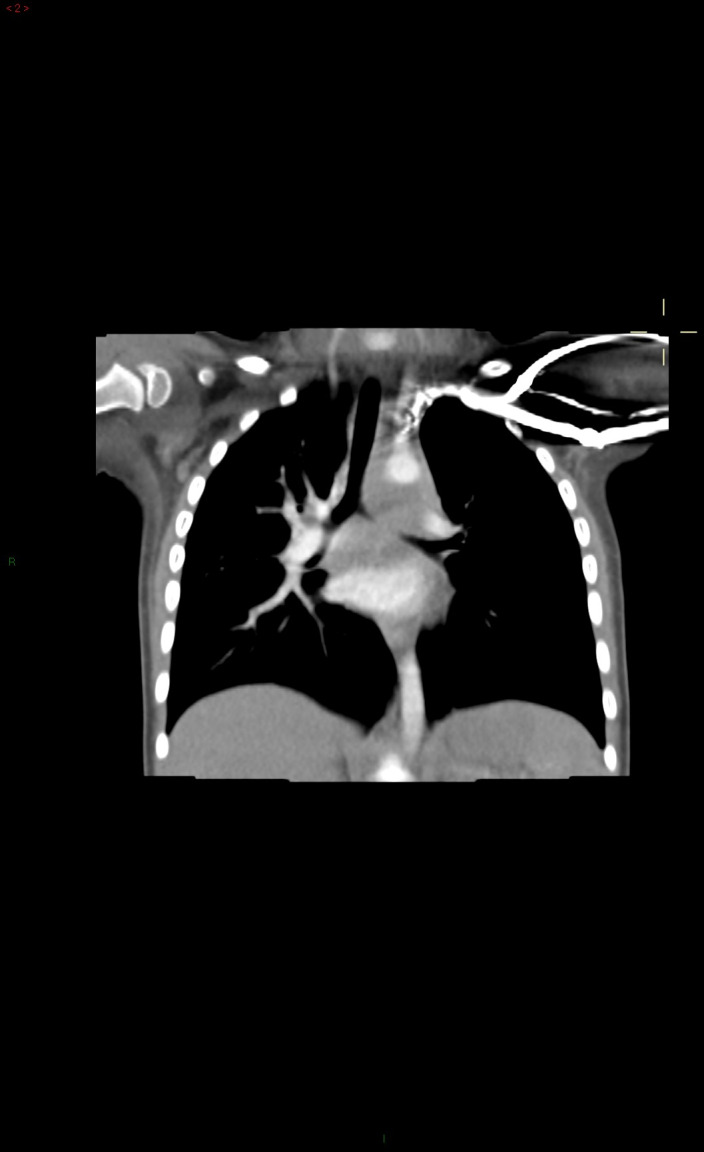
HR‐CT with contrast reveals a large hypodense lesion (34 × 15 × 46 mm) with distinct and smooth margins and fluid density in the posterior mediastinum. This cystic lesion induces the left lung hyperinflation and hypoplasia of left pulmonary artery due to displacement and extrinsic compression of the trachea, left bronchus, and left pulmonary artery
